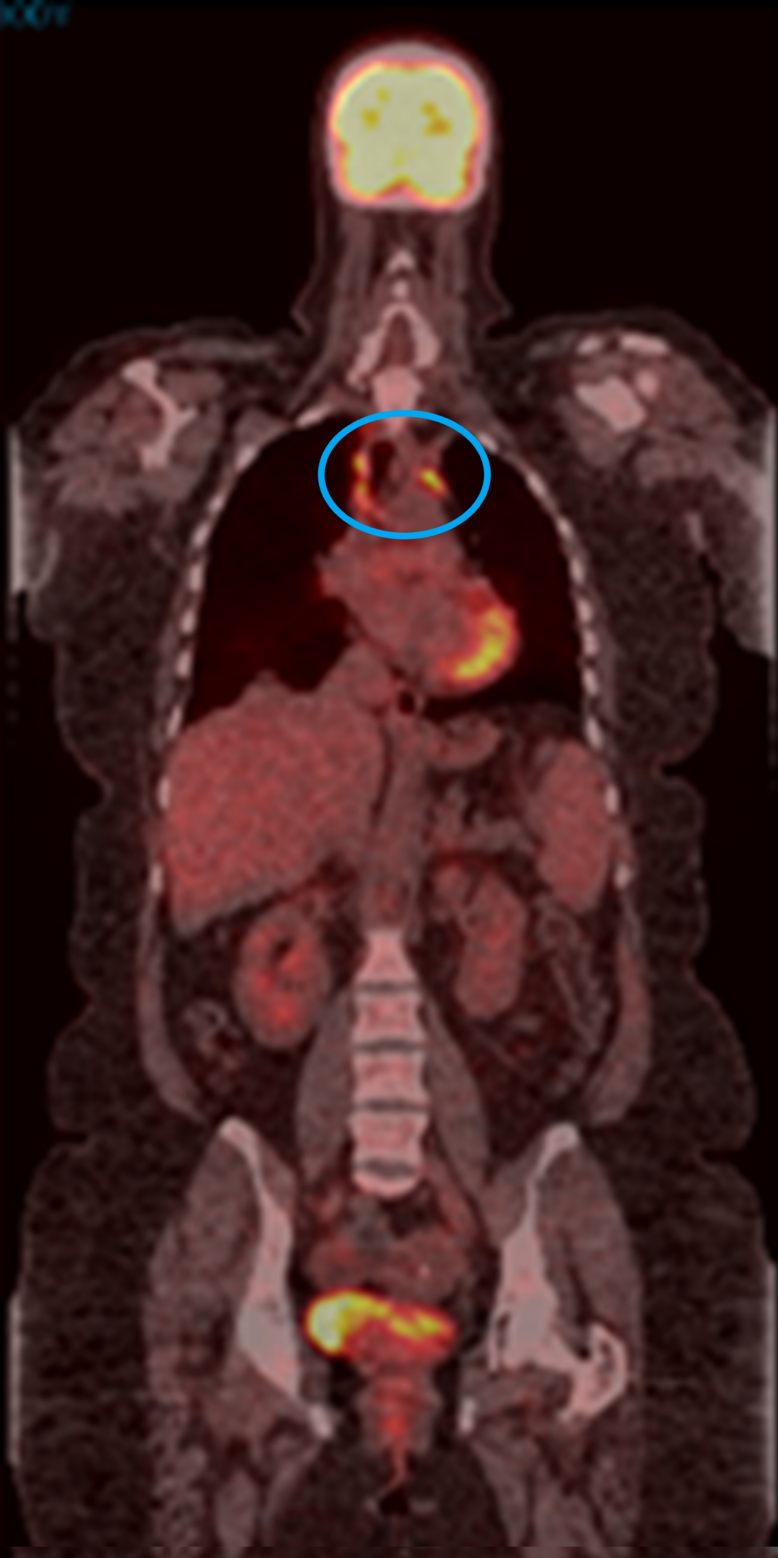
PET/CT scan in September 2017 The image shows uptake of mediastinal lymphadenopathy (blue circle) PET: positron emission tomography; CT: computed tomography
